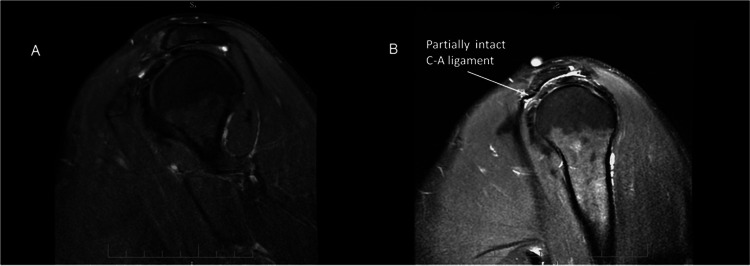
(A) Preoperative MRIs of a patient with type 2 acromion; (B) Postoperative MRI of the same patient showing a partially intact coracoacromial ligament.
